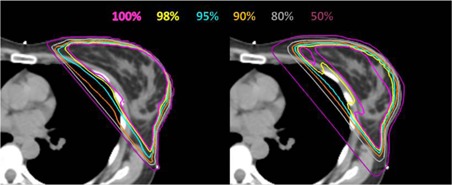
An example of the planned (left) and delivered (right) dose distribution on an axial CT image. The prescription was 267 cGy×16. The mean RTDs are (0.77, 0.36, 0.43, 0.19, −0.59, −1.00), and the SDs of the RTDs are (1.19, 0.66, 0.87, 0.55, 0.27, 0.36).
